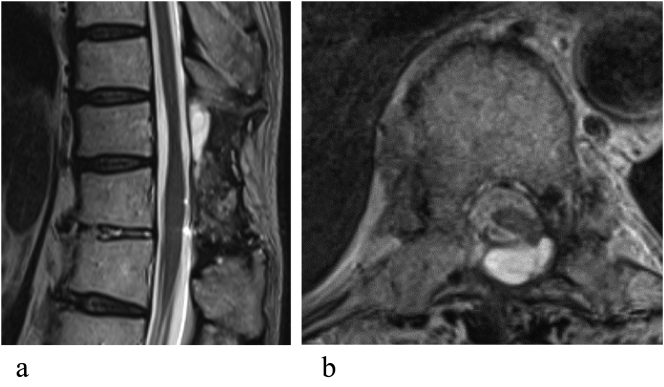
T2-weighted magnetic resonance scans show an extradural cystic lesion associated with laminectomy at T10–11. (a) Sagittal view. (b) Axial view.
